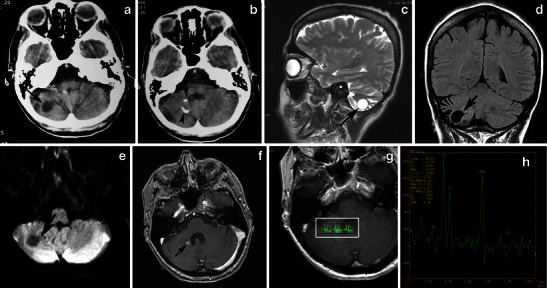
A pilocytic astrocytoma of the right cerebellar hemisphere incidentally discovered during staging in a 45-year-old woman with breast cancer. a An axial CT image shows a well-marginated cyst in the right cerebellar hemisphere (arrow). b An axial CT image in a higher level shows fleck-like calcifications (arrowheads). On sagittal T2-weighted (c), coronal FLAIR (d), and axial diffusion-weighted (e) images, the cyst follows cerebrospinal fluid intensity (arrows). This axial post-contrast T1-weighted image (f) depicts an enhancing focus (arrow). On MRS centred on an enhancing nodule with TE 144-ms (g, h), there is mild elevation of choline/Cr, mild reduction of NAA/Cr, and a small lactate peak
